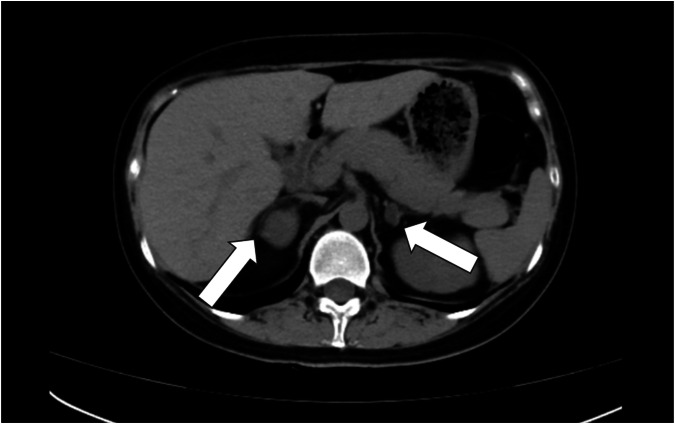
CT imaging of the adrenal glands shows masses on both sides. ) Right adrenal mass, with a slightly hypodense lesion measuring 3.3 × 2.2 cm in its largest cross-section; ) left adrenal mass, with a slightly hypodense lesion measuring 1.3 × 0.8 cm in its largest cross-section
